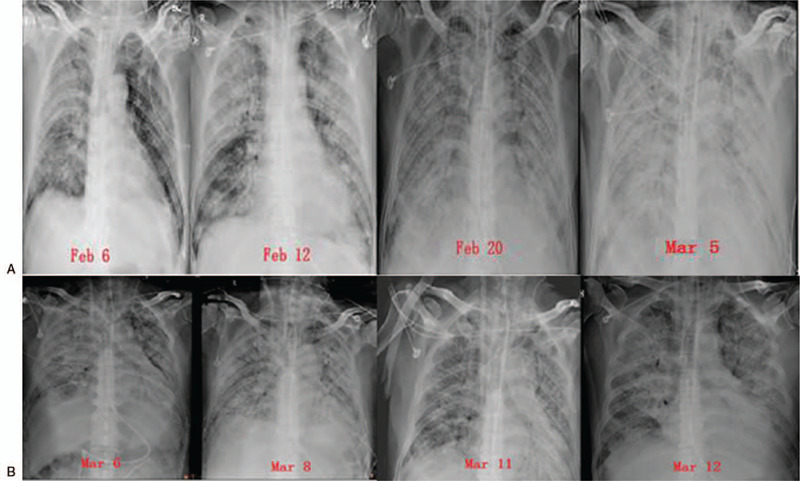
Chest X-ray images of the critically ill patient with acute respiratory distress syndrome from COVID-19 after receiving mechanical ventilation with a higher level of PEEP at the early stage of disease (A) and with a lower level of PEEP at the late stage of disease (B).
